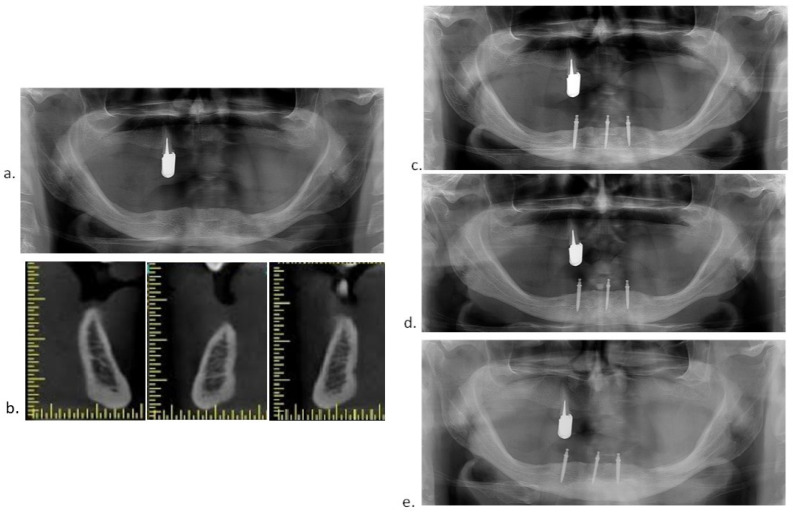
(a) Preoperative panoramic radiograph; (b) preoperative CBCT; (c) panoramic radiograph after insertion of 3 MDIs; (d) panoramic radiograph obtained at the 3-year follow-up examination; (e) panoramic radiograph obtained at the 5-year follow-up examination.
